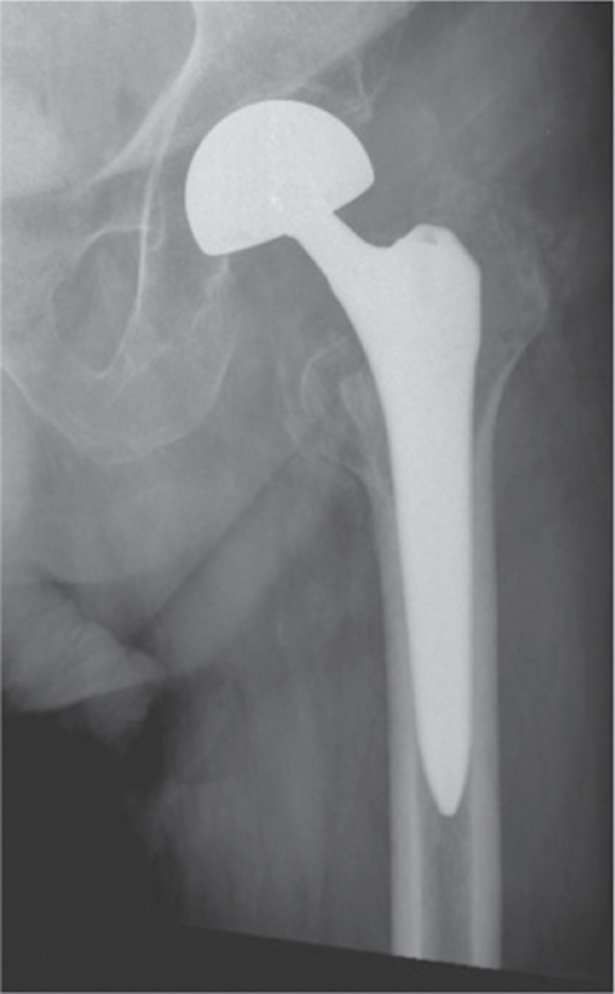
X-Ray view of the standard-stem cementless bipolar hemiarthroplasty (SSCBH).
